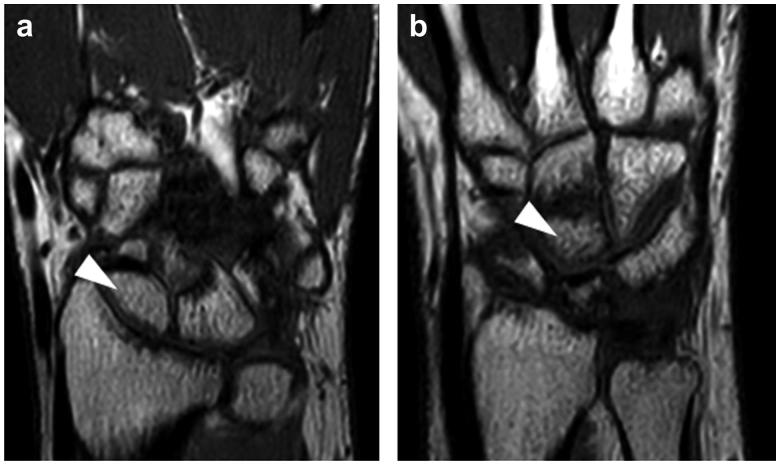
Magnetic resonance imaging (MRI) of the hand. T1-weighted MRI images showing proximal bone chips in both the scaphoid (a) and capitate (b) in high brightness. No impeded blood flow or necrosis was observed.
